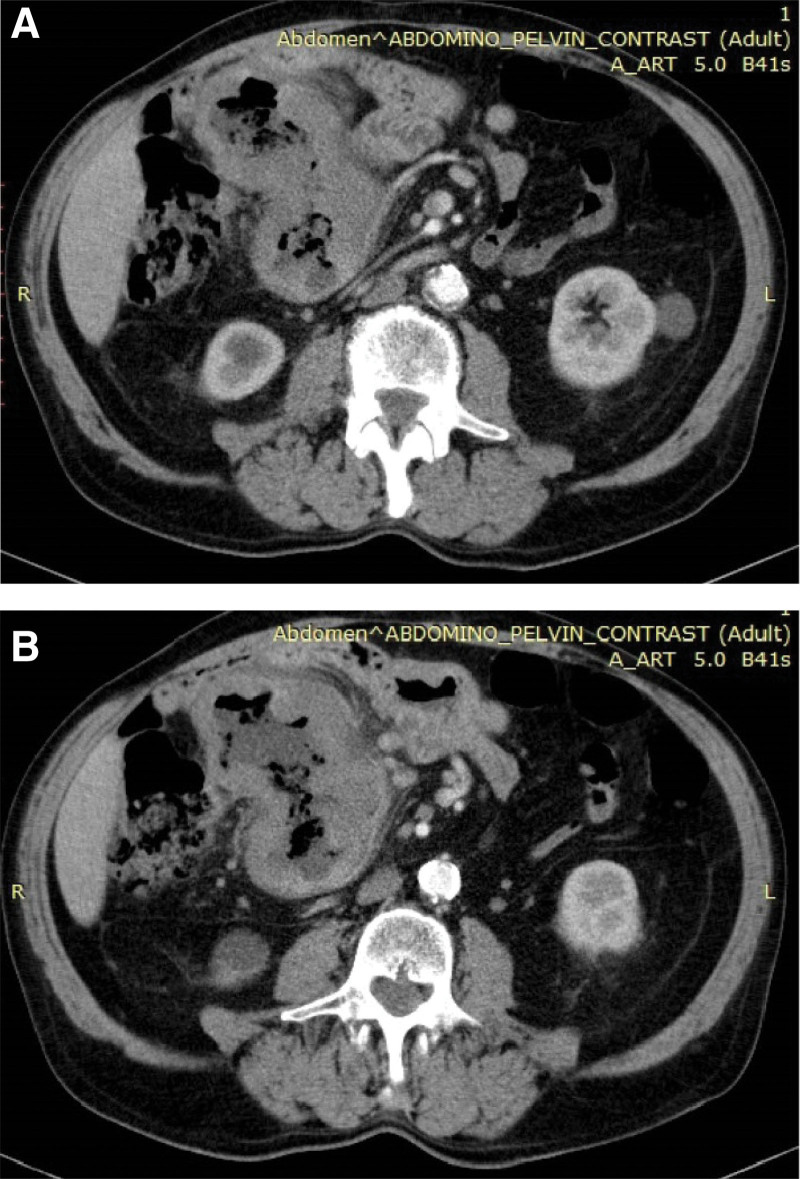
Abdominal computed tomography (CT) scan at the first diagnosis, with intravenous administration of contrast material. (A) The image demonstrates an ileal loop with thickened, iodophilic, irregular walls, located adjacent to the ascending colon, with infiltration of adjacent adipose tissue. (B) The image shows adenopathies and mesenteric adenopathic blocks (maximum 3.9 × 2.6 cm).
